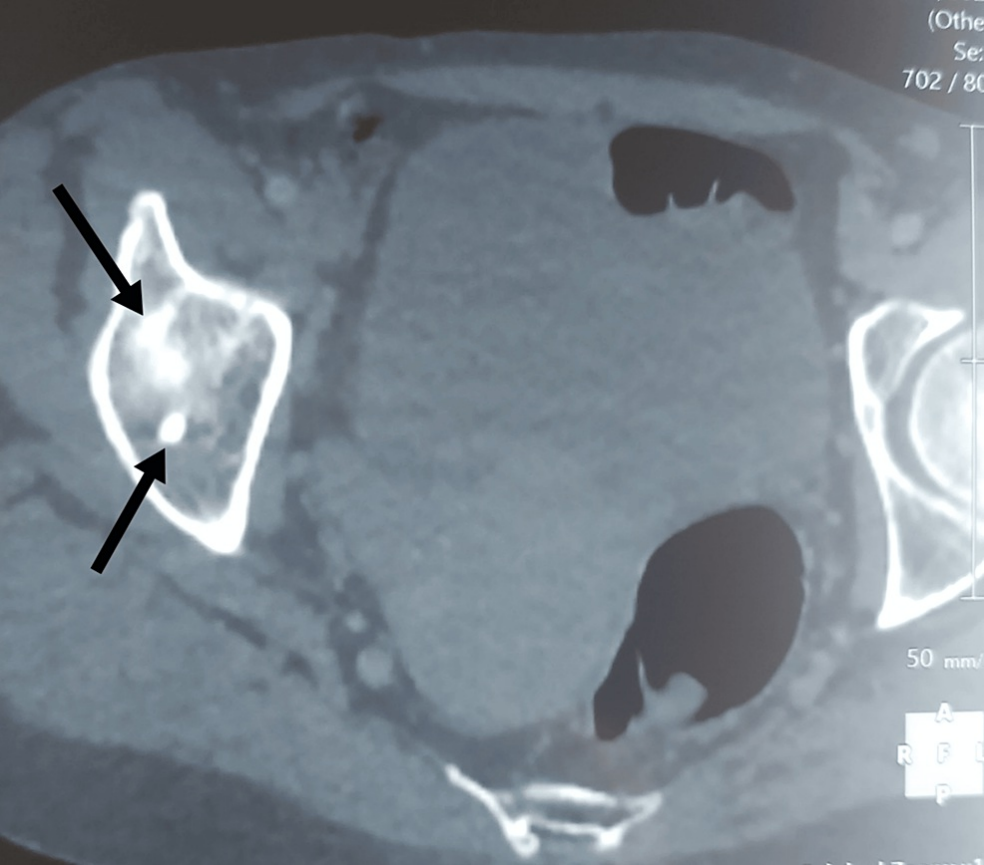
CT scan of the pelvis shows densely sclerotic bone lesions in the right acetabulum (black arrows). CT: computed tomography.
